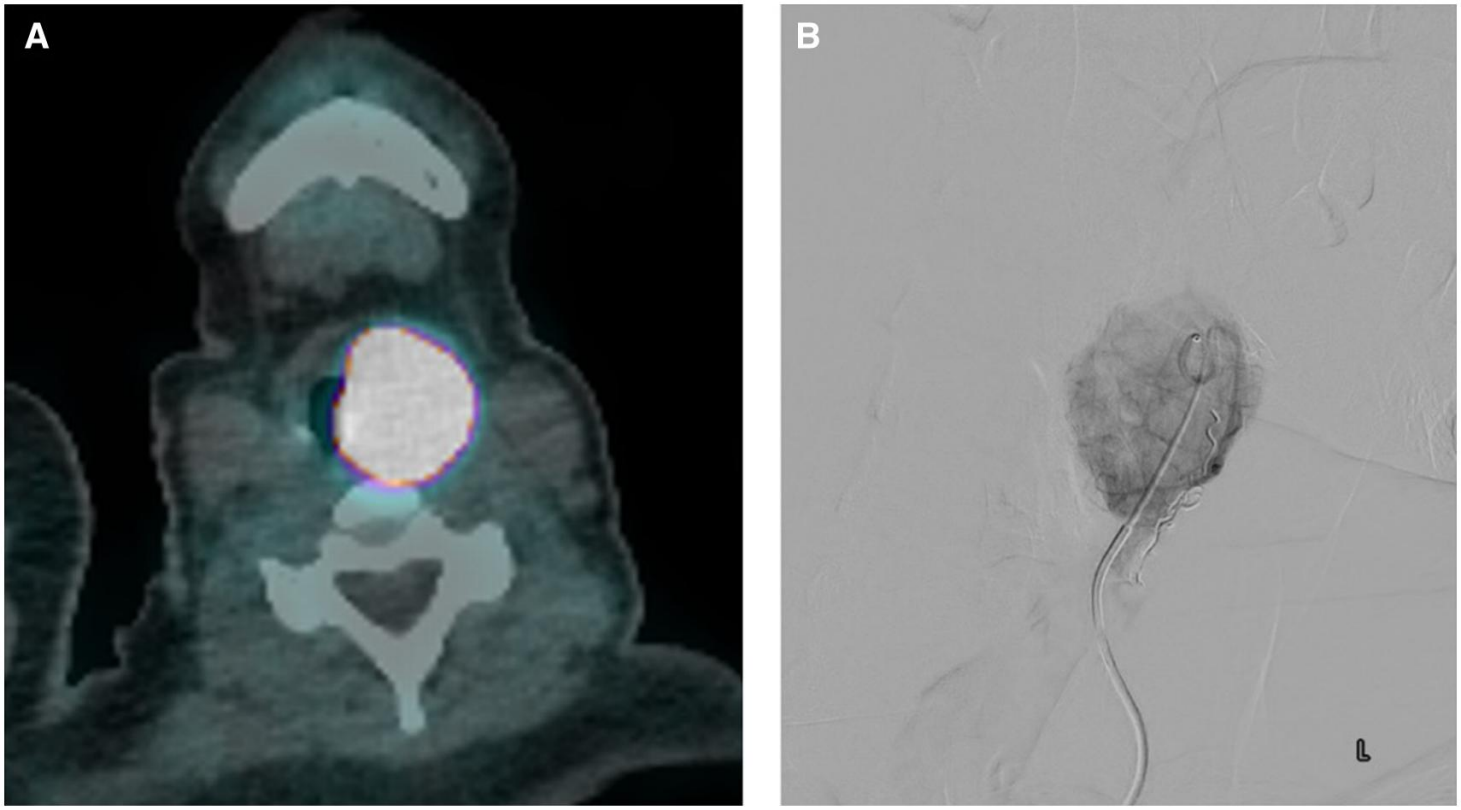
Left supraglottic (aryepiglottic fold) NET. (A) Gallium-68 DOTATATE PET-CT and (B) digital subtraction angiography. MRI appearances were non-specific; however, the intense radiotracer uptake and highly vascular nature of the lesion are more specific for a NET.
